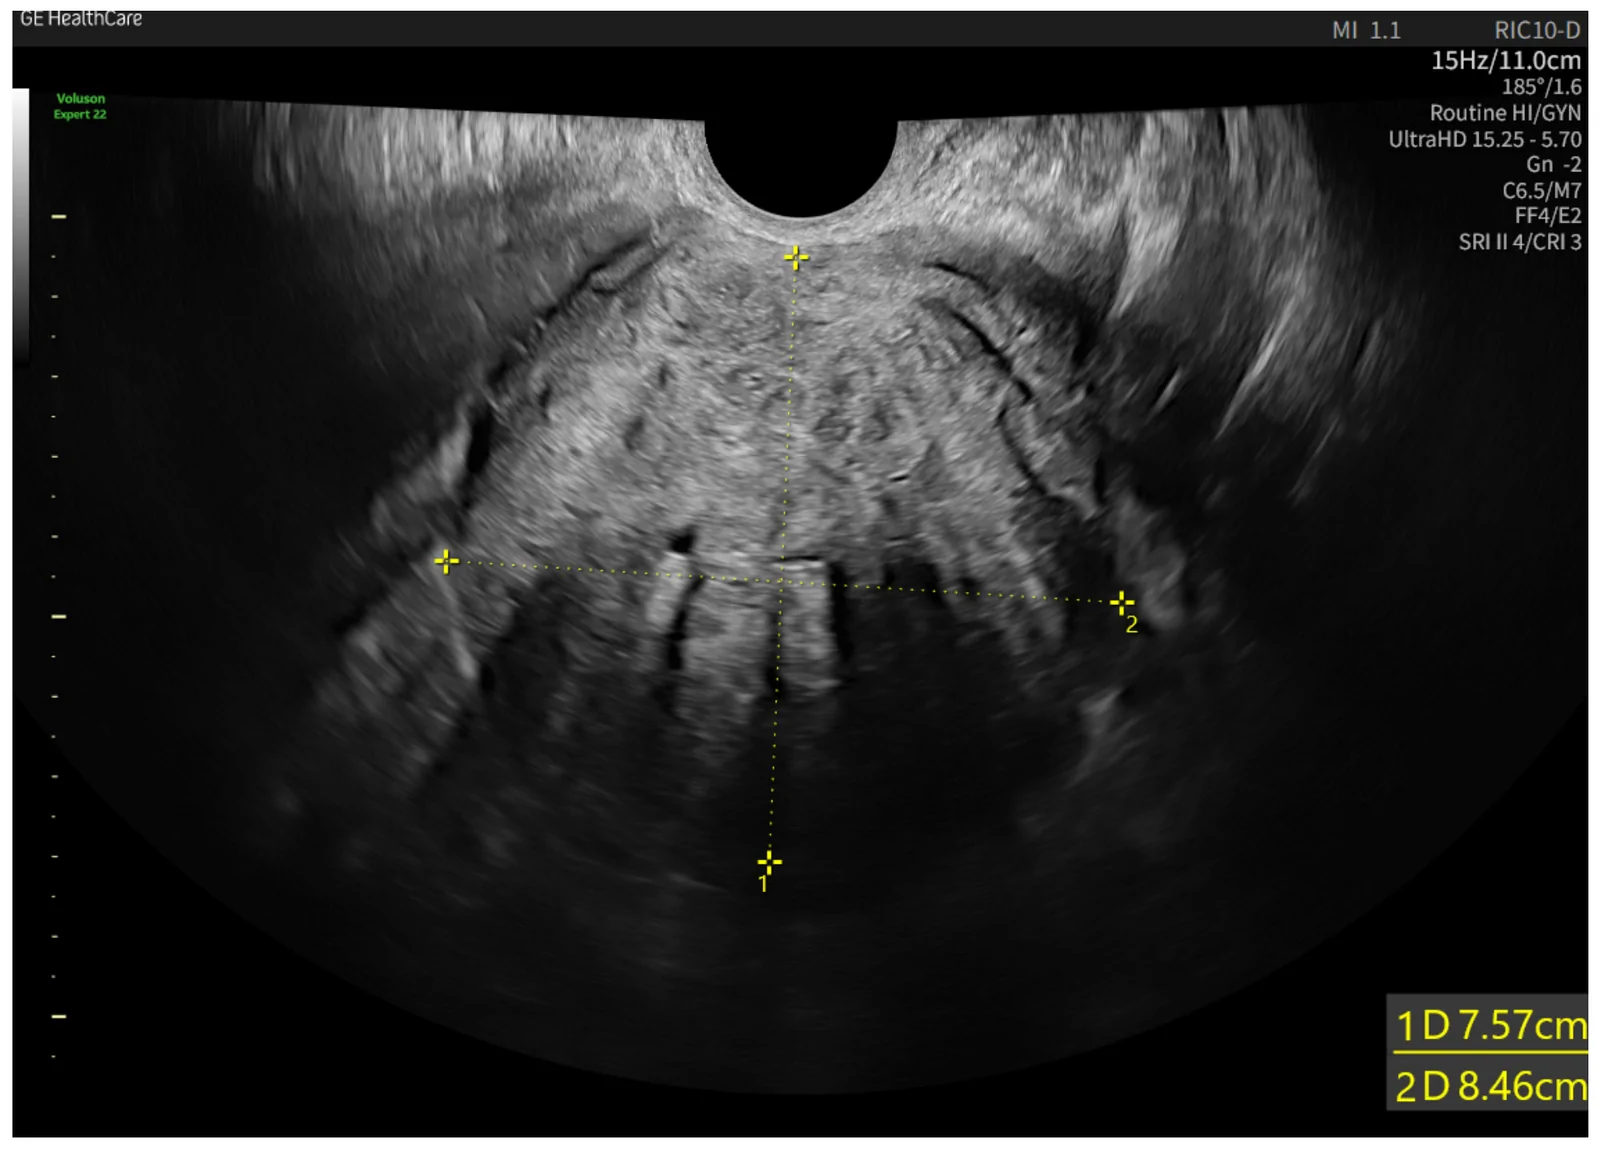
Transvaginal ultrasound appearance of the anterior uterine wall lesion.
